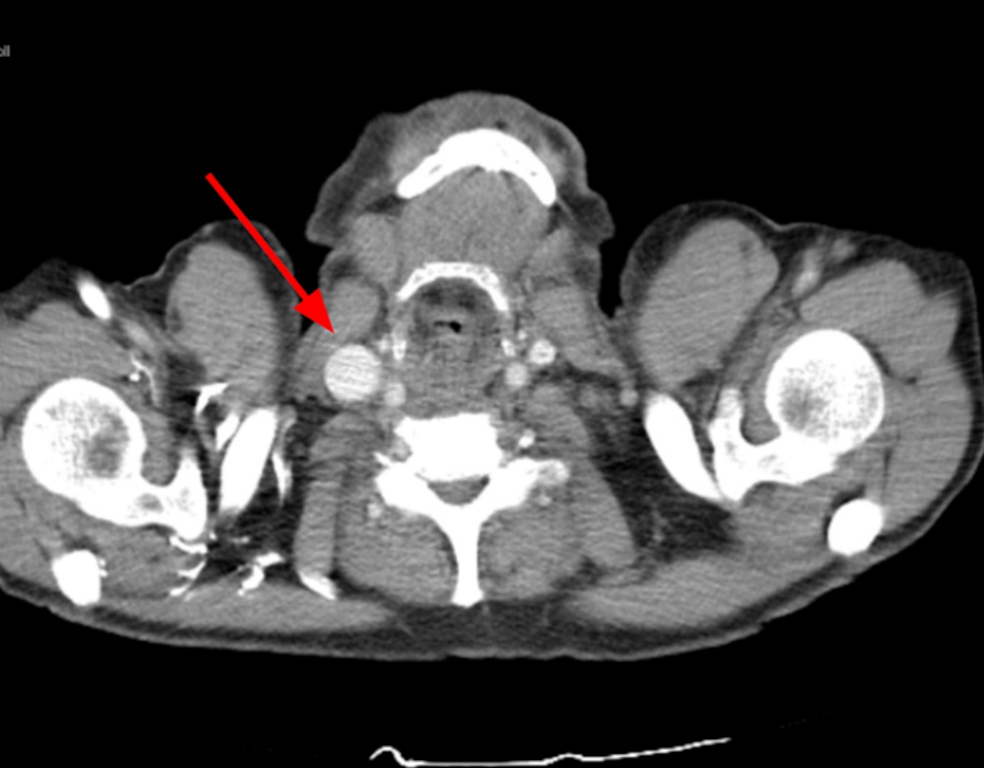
Computed tomography chest showing dilation of the right internal jugular vein (arrow) in comparison to the left internal jugular vein.
